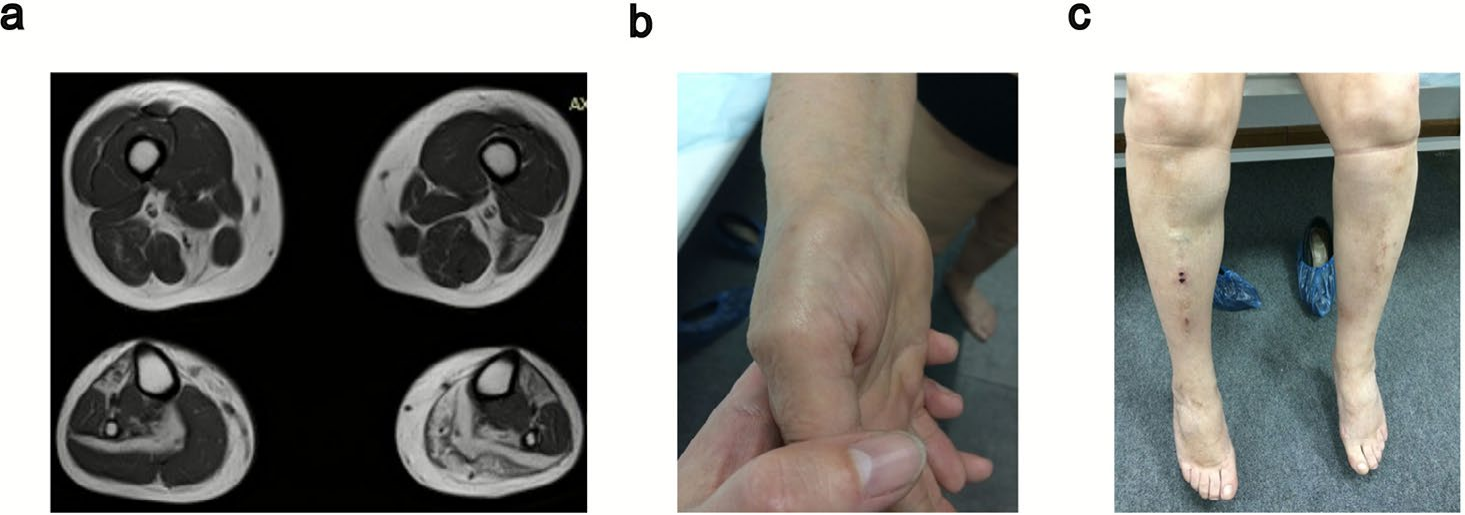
Main clinical findings in Patient 2. (a) Muscle MRI of the lower limbs showing less severe changes in the thigh with fatty degeneration on the right in Adductor magnus and semitendinosus, biceps femoris on the left side, and the typical early changes observed in juvenile onset recessive distal titinopathy with severe fatty replacement in soleus and anterior compartment muscles (in this case, also asymmetric gastrocnemius involvement on the left side). (b) Thenar and mild hypothenar hypotrophy. (c) Distal lower leg weakness.
